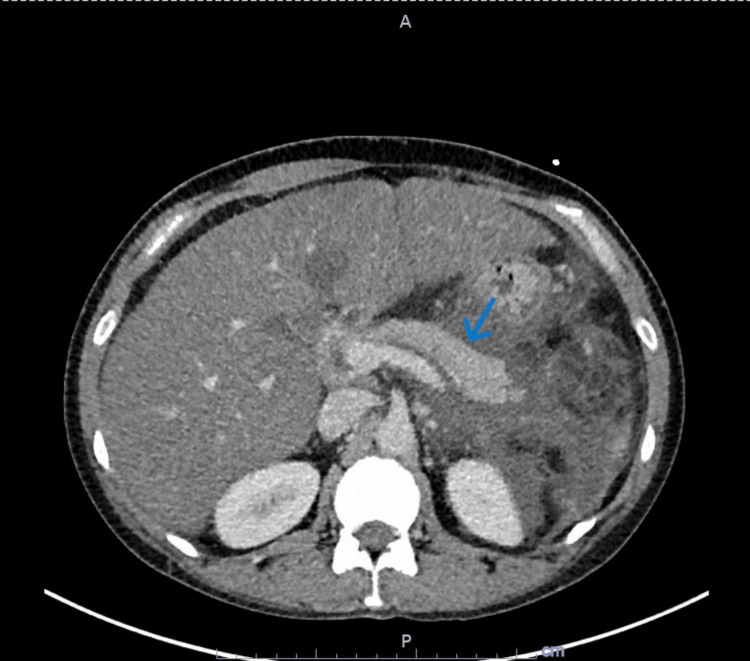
CT abdomen and pelvis with IV contrast Extensive fluid and fat stranding in the left abdomen, including in the lesser sac, which is compatible with clinically suspected pancreatitis. There is no loculated fluid collection identified. The pancreatic parenchyma is enhancing throughout without evidence of pancreatic necrosis. IV: Intravenous
